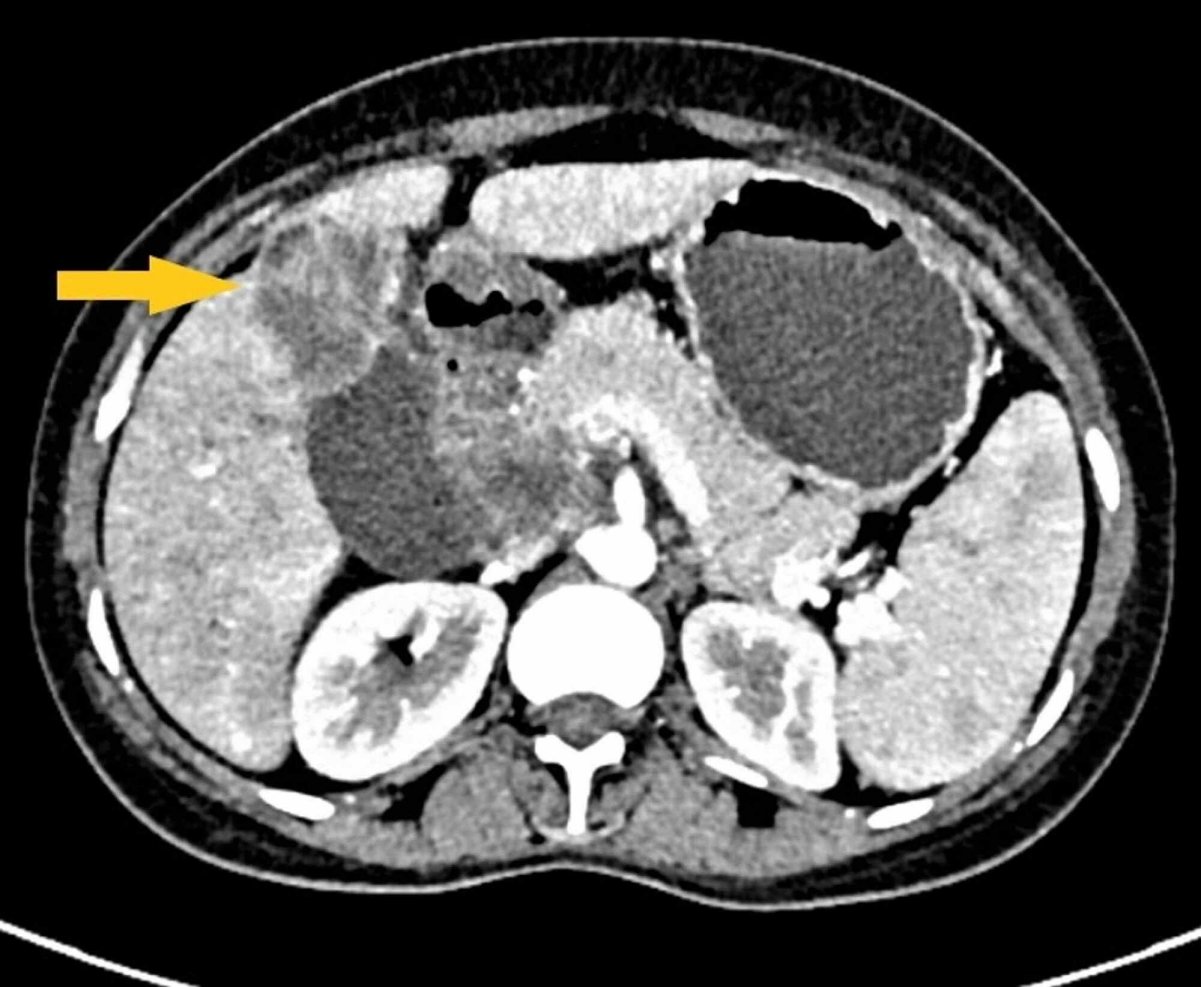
CECT abdomen showing an exophytic gallbladder mass infiltrating liver parenchyma (yellow arrow) CECT: contrast-enhanced computed tomography.
